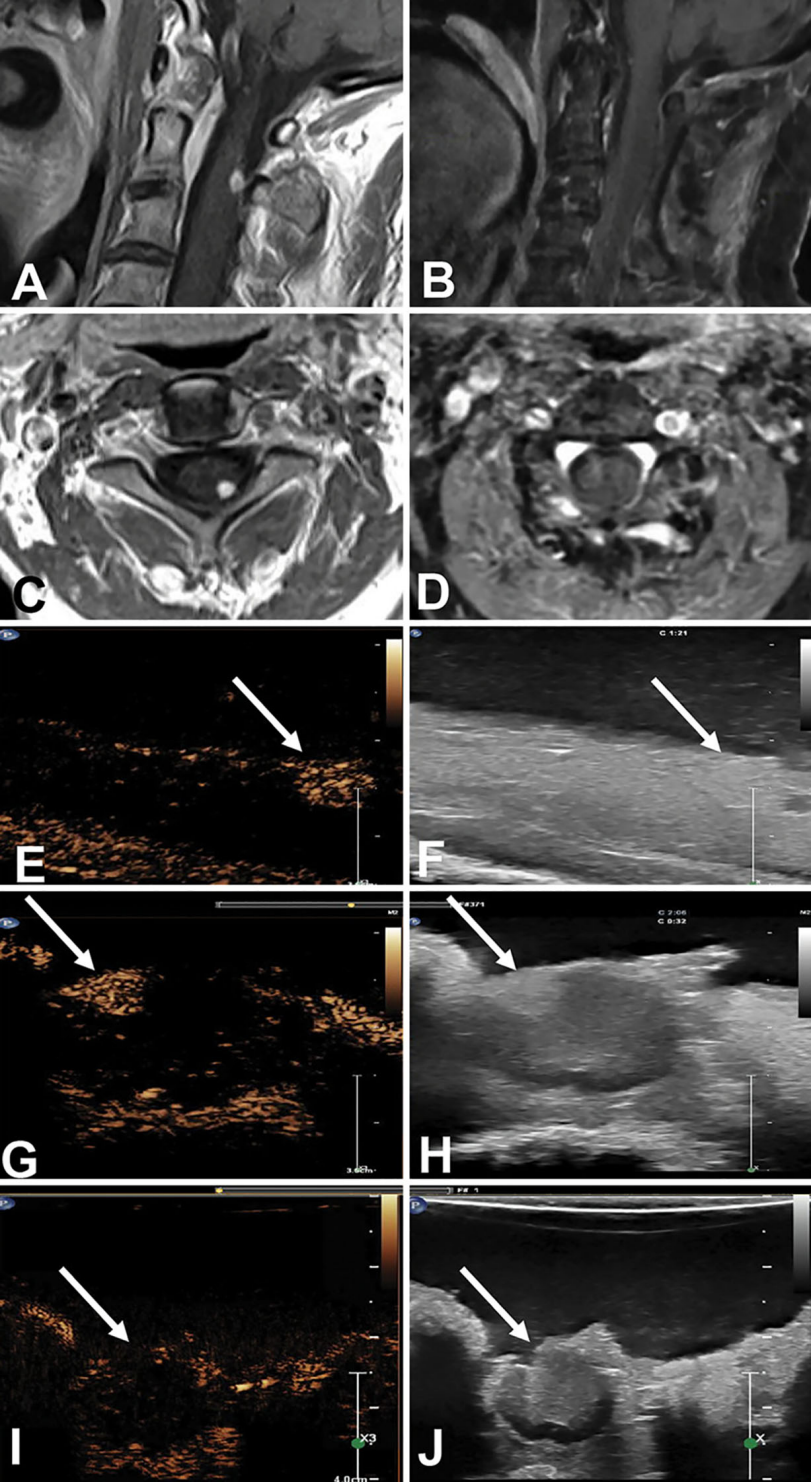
Comparison of standard B-mode ultrasound and Contrast-Enhanced Ultrasound (CEUS) in a spinal intramedullary tumor. (A-D) Preoperative MRI and B-mode imaging. (E, G, I) CEUS imaging reveals a rapidly enhancing, well-circumscribed vascular lesion (arrow), allowing for precise differentiation from the surrounding spinal cord, whereas (F, H, J) standard B-mode imaging demonstrates a hyperechoic lesion with less distinct margins. Reprinted from Barkley et al. (2021) [9], distributed under the terms of the Creative Commons Attribution-NonCommercial-NoDerivatives 4.0 International License (CC BY-NC-ND 4.0).
